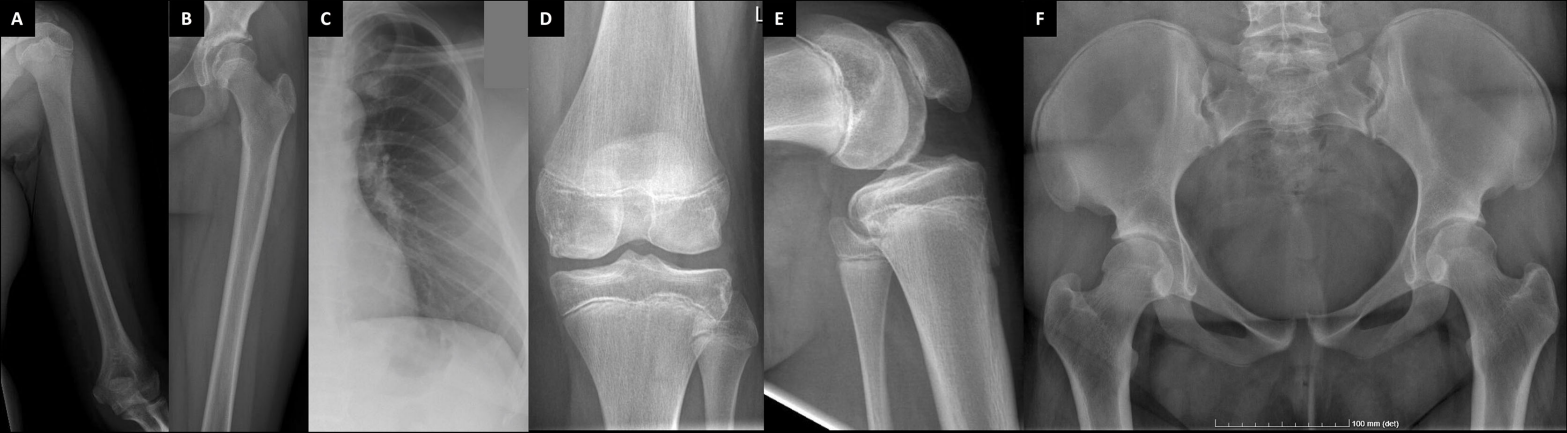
Plain radiographs of the female patient with a novel *TBX2* missense variant p.Thr300Met. The index patient (III-1) at age 11 (A–C) and age 24 (D–F). Images show slender diaphyses in long-bones and ribs, abnormal shaping in pelvic bones and degenerative changes on articular surfaces in hip and knee joints.
